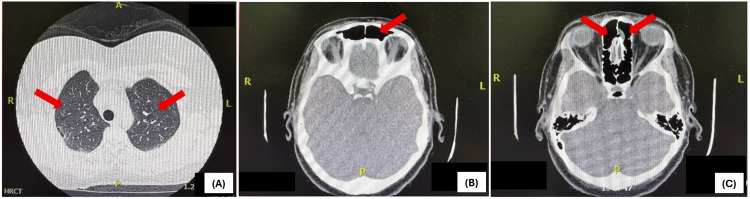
Imaging Findings From Past Medical History (A) HRCT showing bilateral emphysematous changes consistent with COPD. (B) Paranasal sinus imaging demonstrating mild left frontal sinusitis. (C) Paranasal sinus imaging demonstrating mild bilateral ethmoid sinusitis. HRCT: High-Resolution Computed Tomography; COPD: Chronic Obstructive Pulmonary Disease
